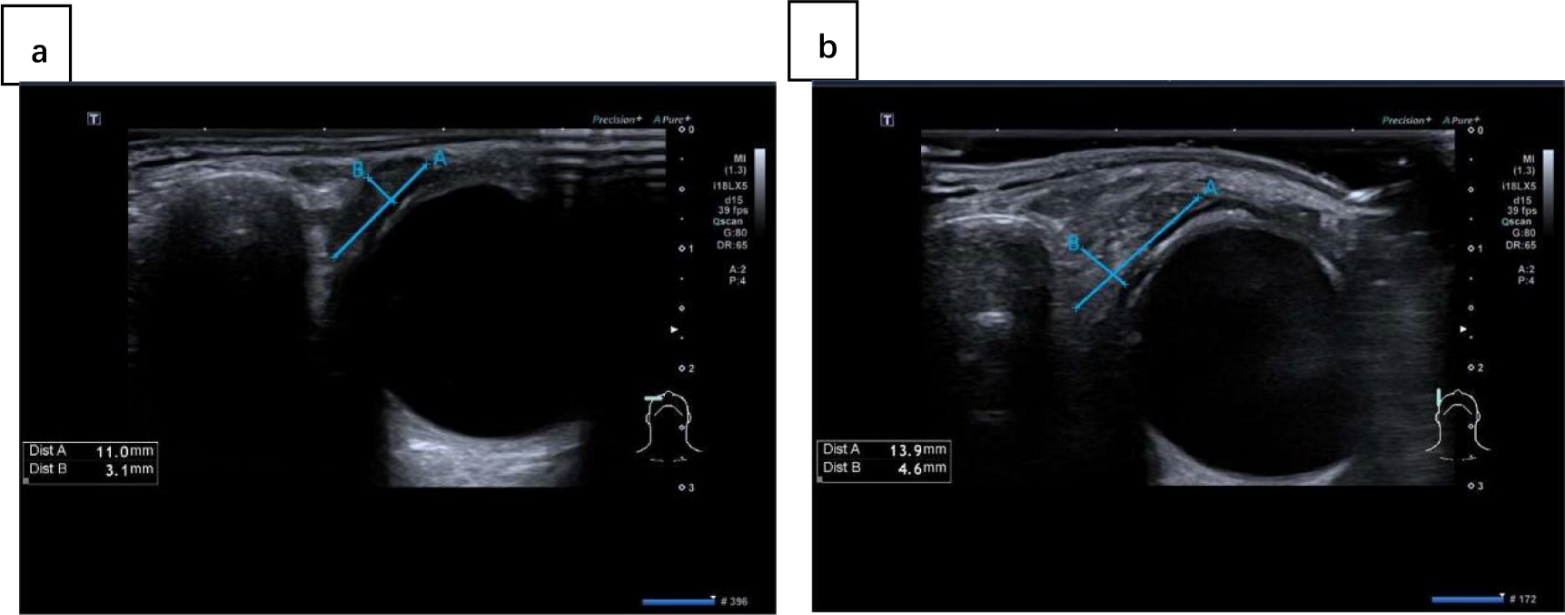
The ultrasound images of lacrimal gland in different planes: (A) transverse plane; (B) vertical plane. Line A represents the long diameter and line B represents the thick diameter.
